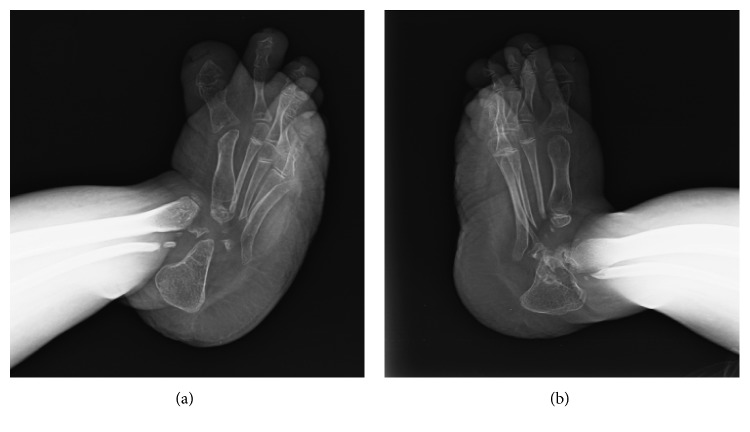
Radiographs of right (a) and left (b) ankles demonstrating the osteolysis of tarsal bones, distal tibial epiphysis, the proximal end of metatarsal bones, and the distal end of fibula. Severe cortical thinning and osteopenia of all bones as well as bowing of both tibias were observed.
